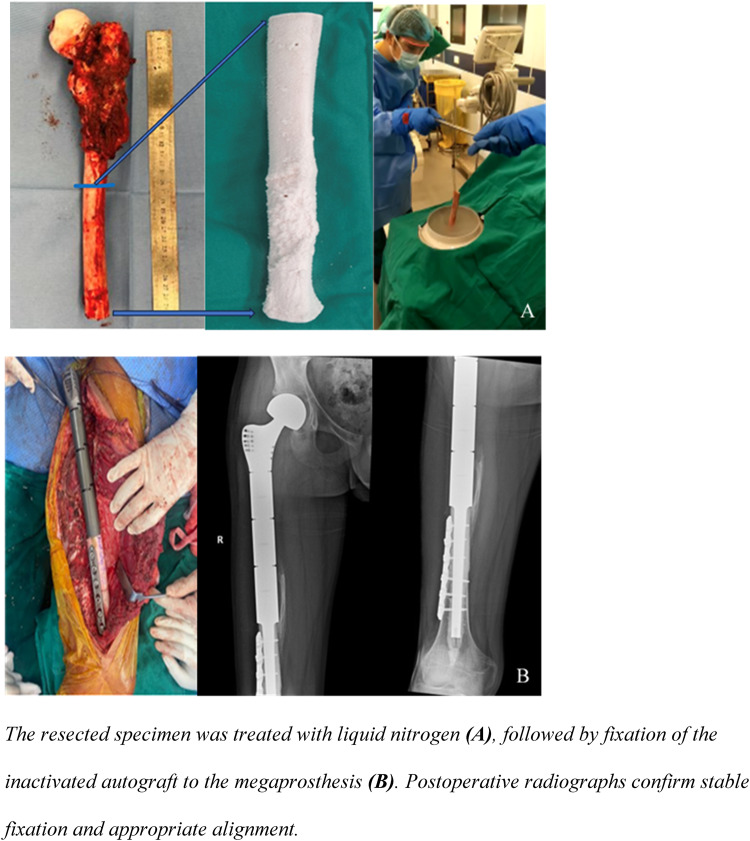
Intraoperative and postoperative radiographs demonstrating reconstruction of a proximal femoral tumor using a liquid nitrogen-treated autograft combined with a megaprosthesis
